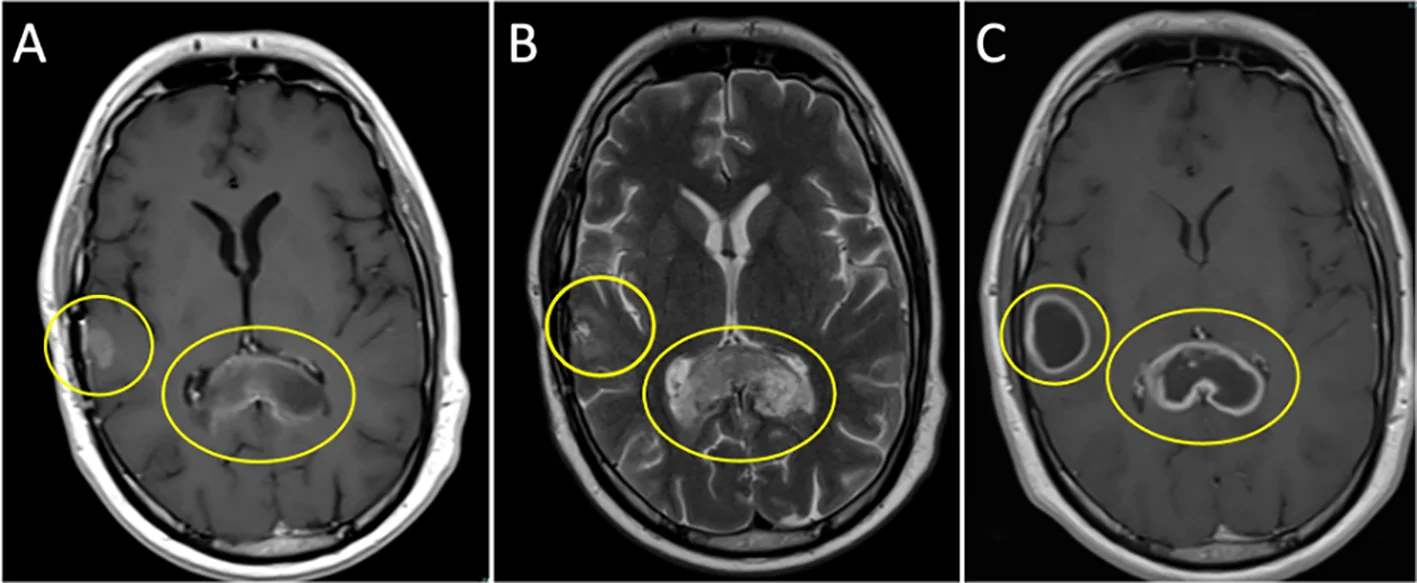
Cranial MRI (A–C) showing multifocal GBM with two supratentorial intra-axial lesions. One was located in the right temporo-opercular region, ovoid in shape, measuring 22.9 mm in latero-lateral diameter, 28.7 mm in anteroposterior (AP) diameter, and 25 mm in craniocaudal diameter. An additional bilateral hemispheric lesion is also present, with its epicenter in the splenium of the corpus callosum, showing moderate peripheral enhancement consistent with a cystic/necrotic lesion, measuring 48.4 mm in latero-lateral diameter, 23.7 mm in AP diameter, and 20.4 mm in craniocaudal diameter. Yellow circles indicate the right temporo-opercular lesion and the bilateral lesion centered in the splenium of the corpus callosum.
